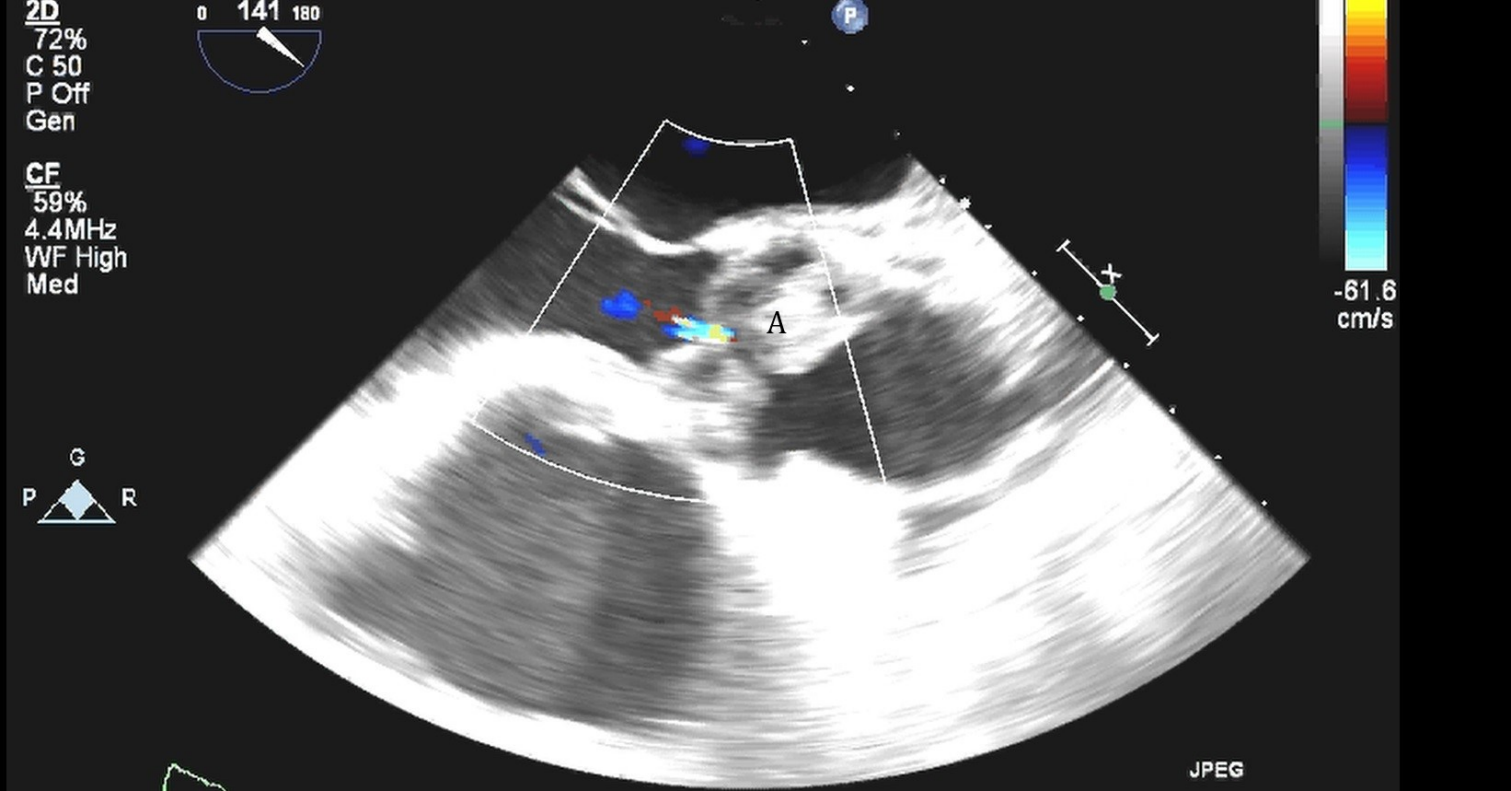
Transesophageal long axis image demonstrating a large aortic
